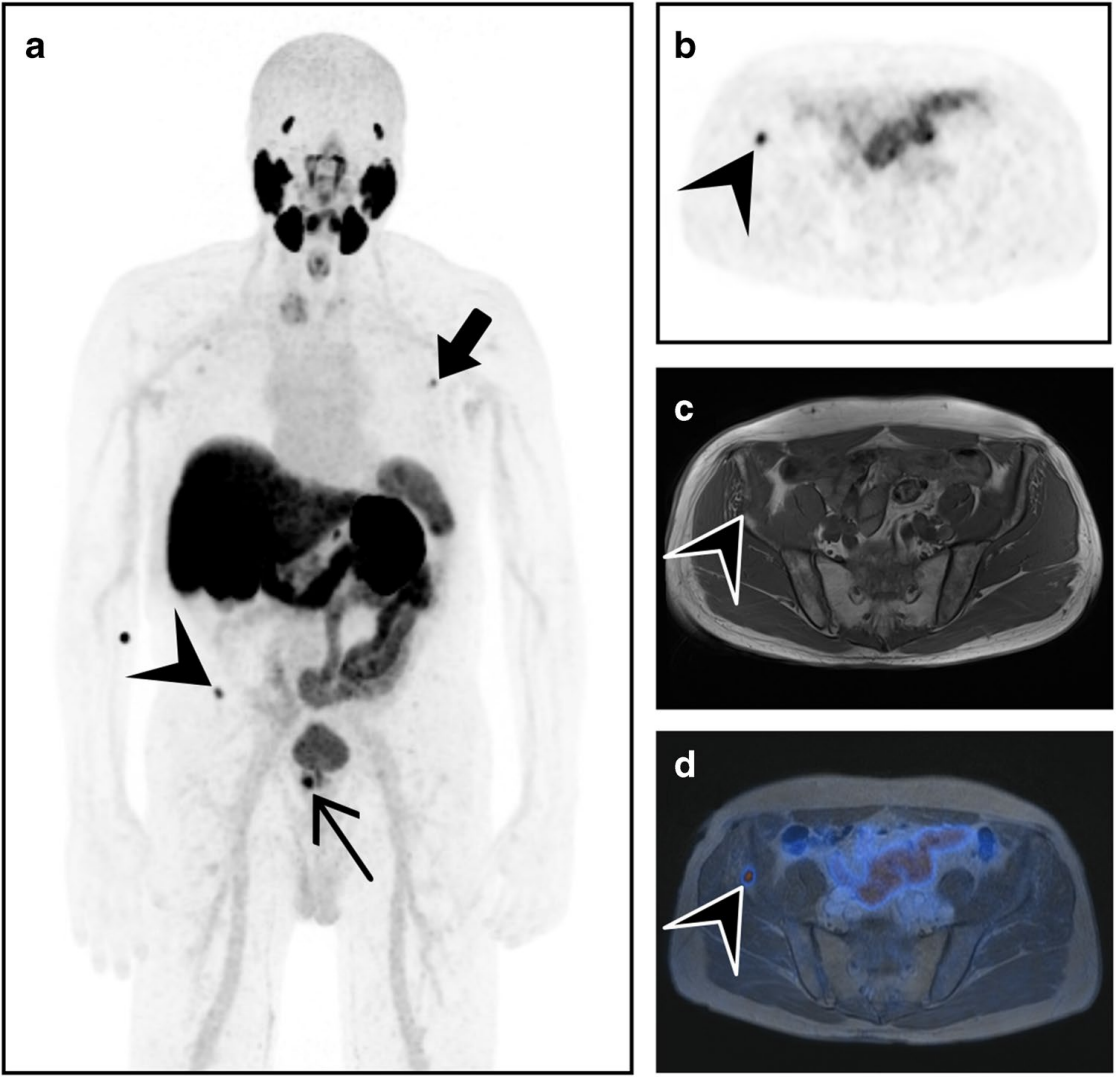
Pretherapeutic [^18^F]-PSMA-1007-PET/MRI staging examination of a 71-year-old patient with prostate cancer, tumor stage pT3b pN0, ISUP 4, and initial PSA of 24.4 µg/l. a A whole-body maximum intensity projection showing the PSMA-positive prostate cancer (thin arrow), no lymph node metastasis, an unspecific bone uptake (SUV_max_ 7.0) in one left rib (bold arrow), and a stronger PSMA-positive bone lesion in the right pelvis (SUV_max_ 8.7). b The pelvic bone lesion in PET (arrow head). c A hypointense morphological correlate on T1-weighted imaging. d The lesion on fused PET/MRI (arrow head). After radical prostatectomy, the PSA normalized (several follow-ups with a PSA of < 0.05 µg/l) without further therapy, suggesting that both bone lesions were most likely benign
